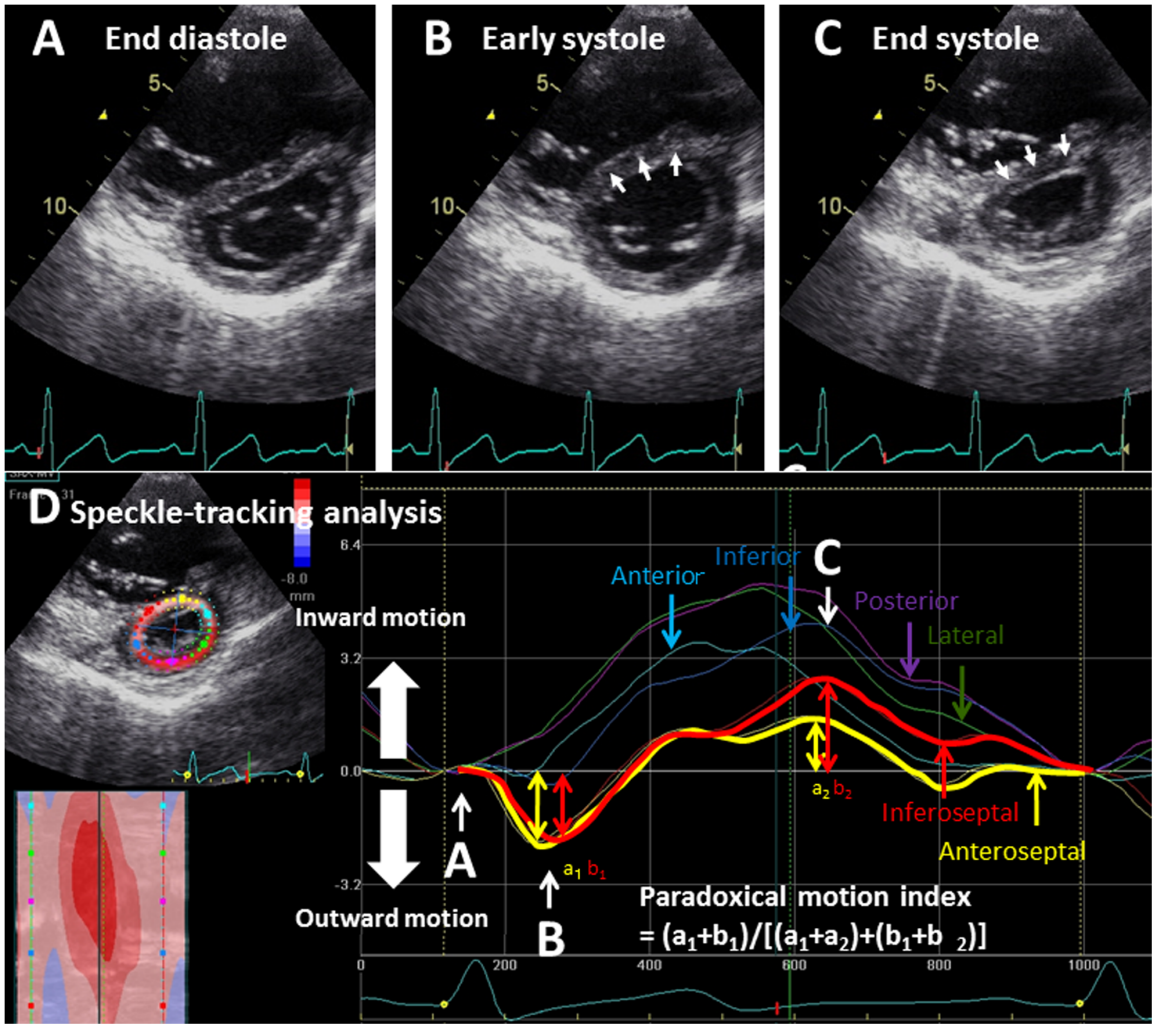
Quantitative assessment of paradoxical motion of the interventricular septum. Panels A, B and C show short axis images of the left ventricle at three cardiac phases of a patient with PH. From the end-diastolic phase (A) to the early systolic phase (B), the interventricular septum moves outward (toward right ventricle) in a paradoxical manner (B, arrows). Subsequently, from early systole (B) to end-systole (C), the interventricular septum moves inward in a manner similar to that of the other segments of the LV wall (panel C, arrows). However, the maximum inward motion was less than that of the other LV wall segments (D). To quantify the paradoxical interventricular septal motion indicated by arrows in panel B, we used speckle-tracking echocardiography by which the left ventricular free walls and interventricular septum were automatically divided into six segments, and each motion pattern was visualized by six lines (D). Yellow and red lines indicate the motion of the anterior and inferior interventricular septum, respectively, during a single cardiac cycle. The dips in the early systolic phase (a1 and b1) indicate their paradoxical motion. For quantitative assessment, the maximum depths of the yellow and red lines (a1+ b1) were added. Next, the entire distance of the two interventricular septal segments [early systole (a1 and b1) and end-systole (a2 and b2)] were added. Then, (a1+ b1) was divided by (a1+ a2+ b1+ b2), and the result was used as the paradoxical motion index of the interventricular septum.
